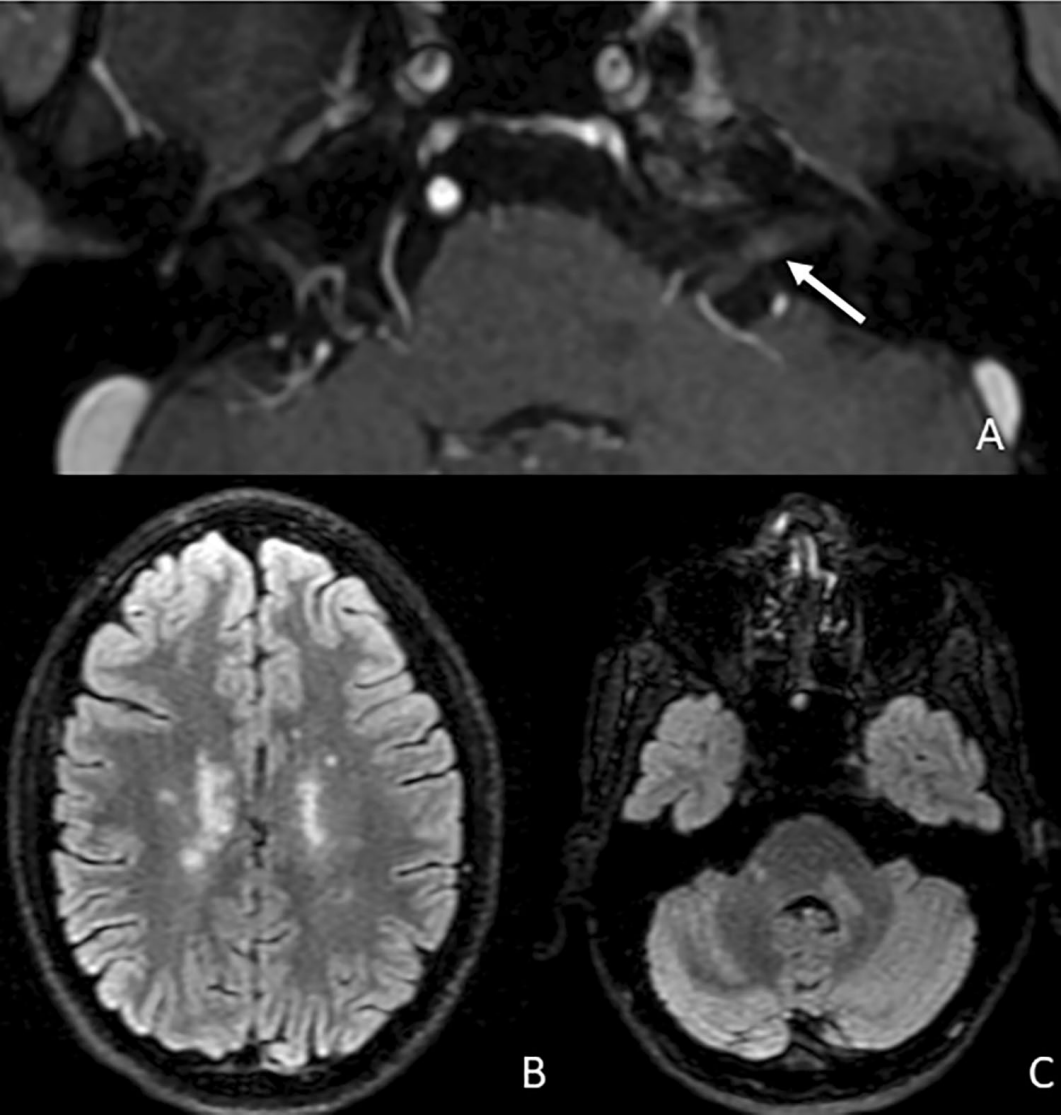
A Axial T1 3D post-contrast image showing a slight linear enhancement of the left VII–VIII cranial nerves in the internal acoustic canal (arrow), in a patient affected by multiple sclerosis with lesions in supratentorial (b. axial fat sat FLAIR) and subtentorial (c. axial Fat sat FLAIR) white matter
